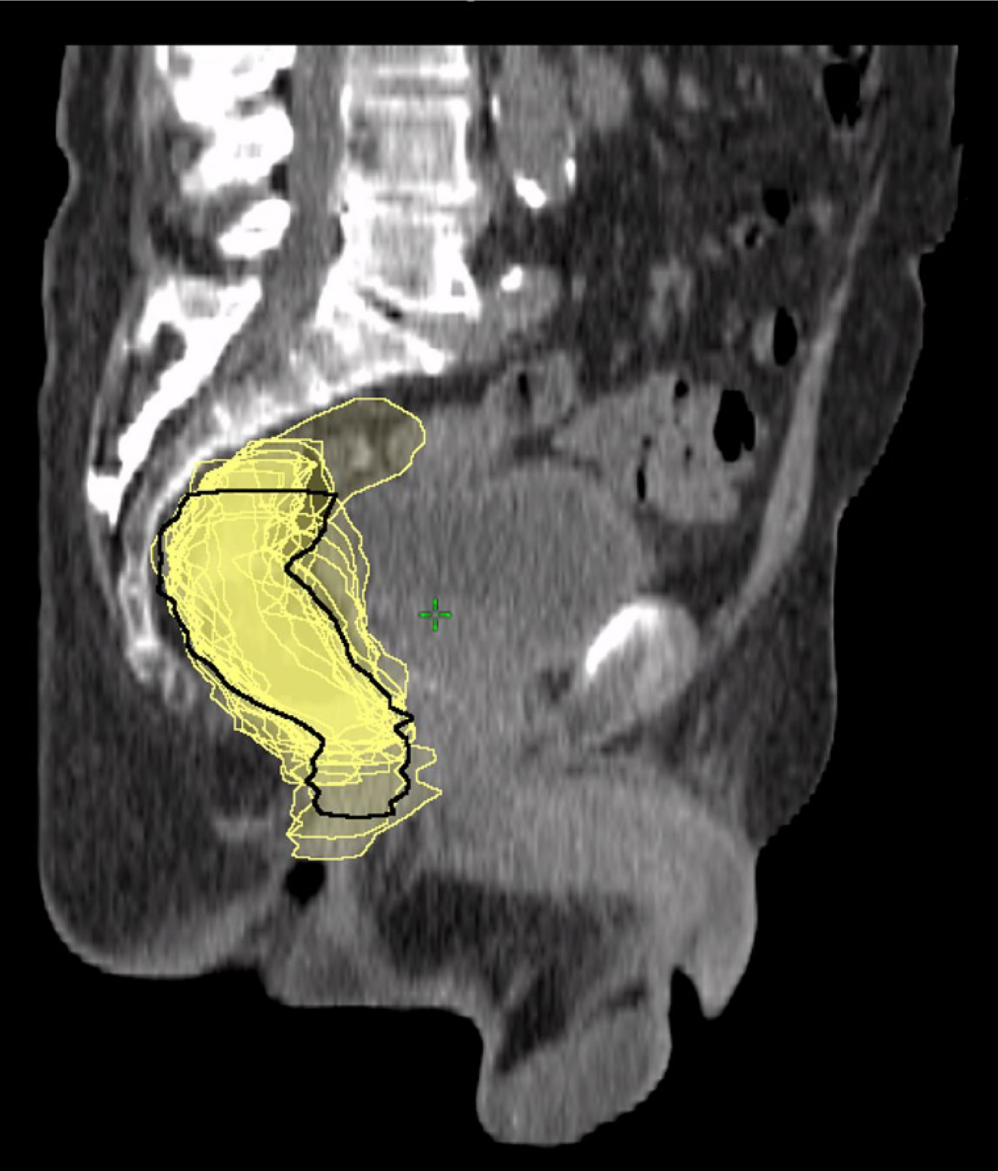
Contoured cone-beam computed tomography (CBCT) structure set overlaid on the planning computed tomography (CT) image. A black contour represents the rectum on the planning CT, while the yellow contours represent the rectum as delineated on the CBCT scans.
